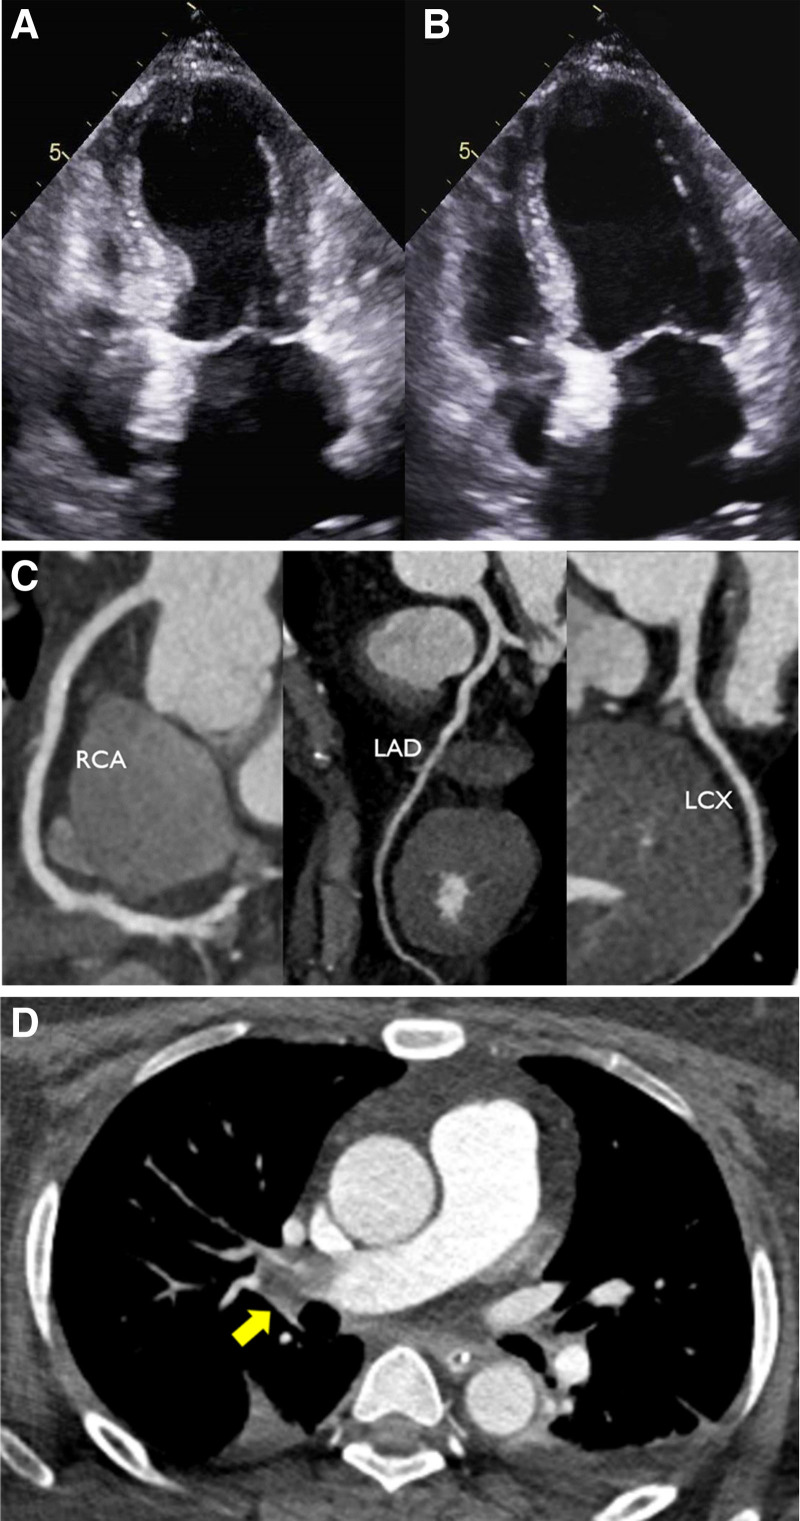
(A, B) The apical-4-chamber view in systole and diastole showing classic apical ballooning with akinesia and hyperdynamic basal segments on echocardiography indicating stress-induced cardiomyopathy. (C) Coronary CT angiography showing normal coronary arteries. (D) Contrast CT showing filling defect (yellow arrow) in right pulmonary artery. CT = computed tomography, RCA = right coronary artery, LAD = left anterior descending coronary artery, LCX = left circumflex artery.
